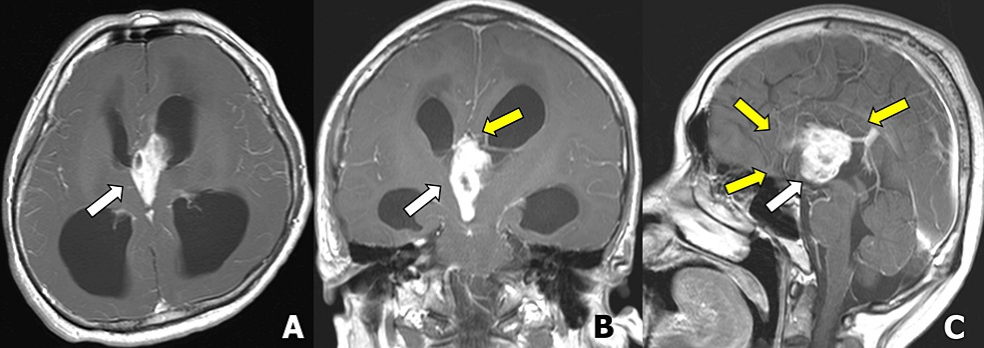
Initial brain ｍagnetic resonance imaging. Contrast-enhanced T1-weighted images show an intraventricular mass in the third ventricle to the anterior horn of the left lateral ventricle (A-C; white arrow) with hydrocephalus. Corpus callosum agenesis is noted in the coronal and sagittal images (B and C; yellow arrows).
